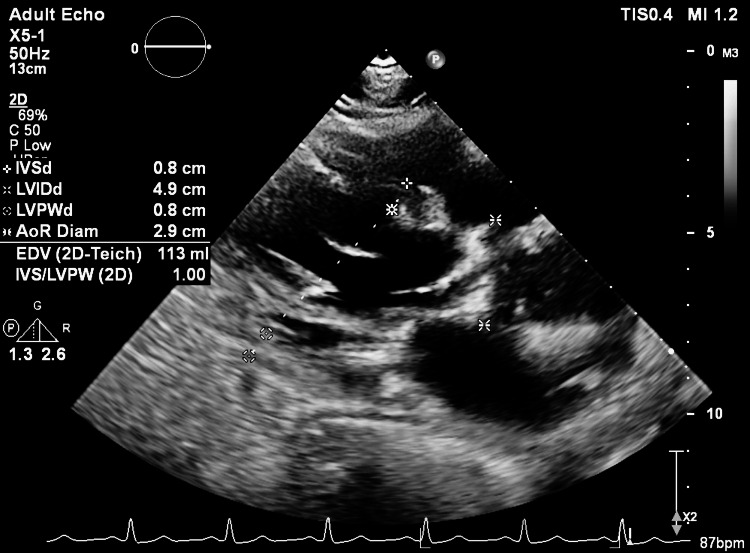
Parasternal long-axis echocardiographic view Demonstrates severe calcification and restricted opening of the aortic valve consistent with critical aortic stenosis.
